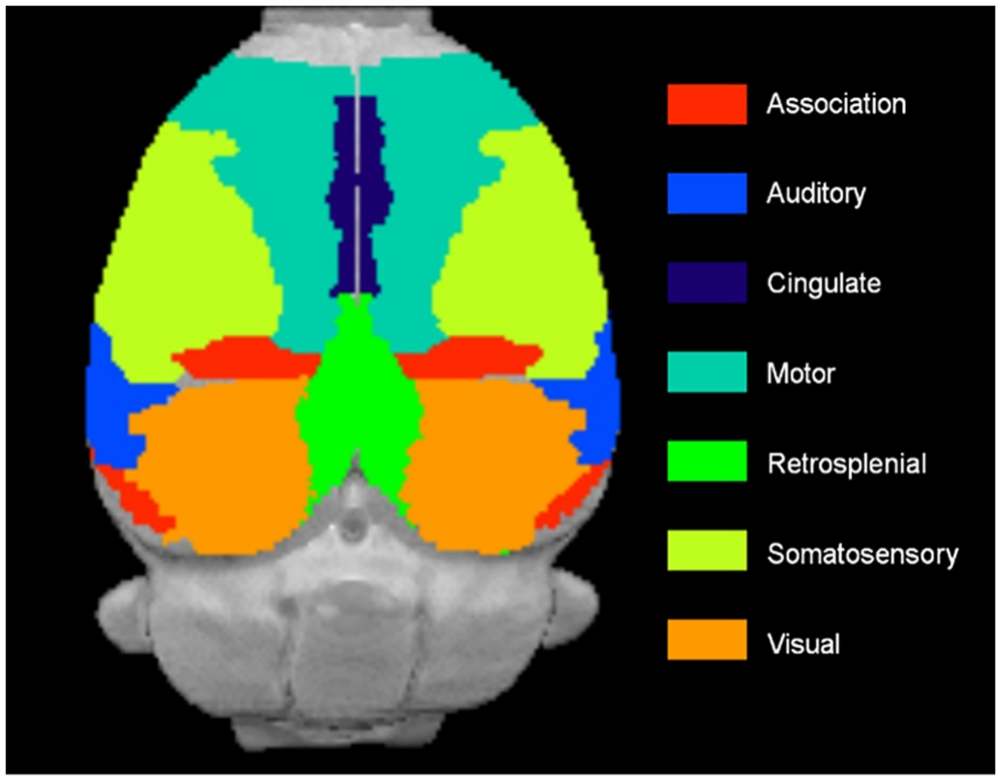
Cortical regions superimposed on the T2-weighted MRI template. Insular region is ventral and not shown in the figure.
